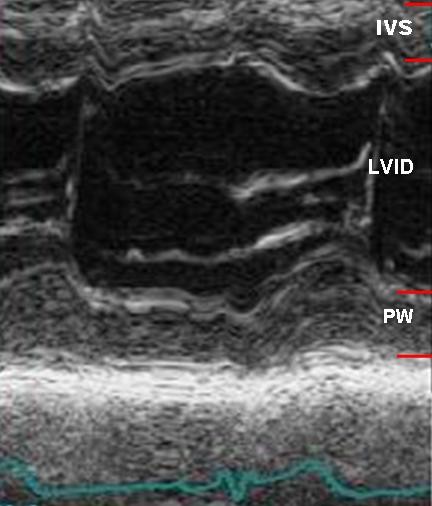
M-mode left ventricular measurements are obtained in parasternal long axis from leading edge to leading edge technique.
